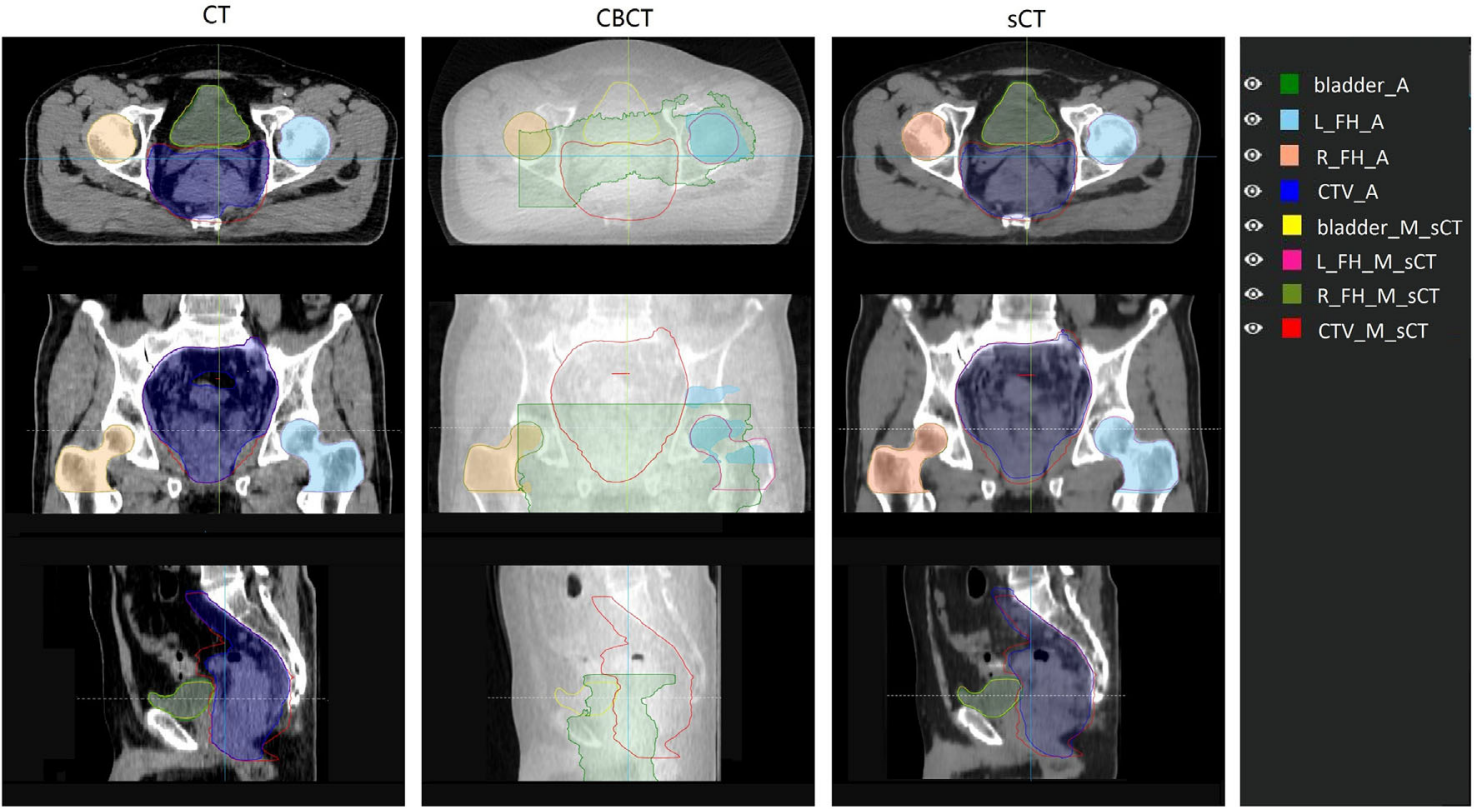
Comparison of auto contour on different image set with the manual contour delineated on sCT. The manual contour was delineated on sCT and then copied to CT and CBCT. Contour name with “_A” suffix represents auto contour. Contour name with “_M_sCT” suffix represents contour manually delineated on sCT. L_FH and R_FH means left femoral head and right femoral head.
